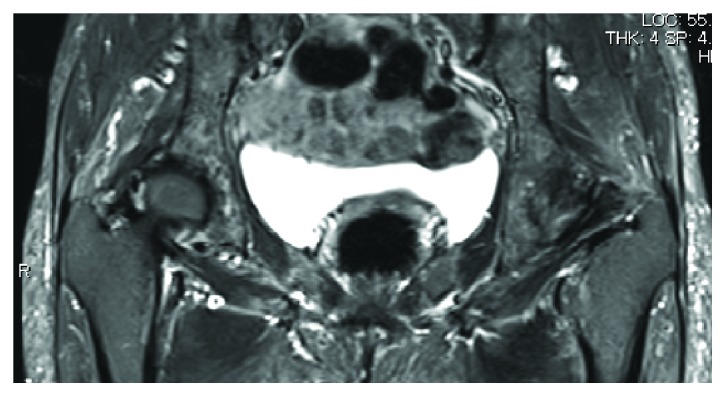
Preoperative magnetic resonance imaging. No abnormal findings are observed.
